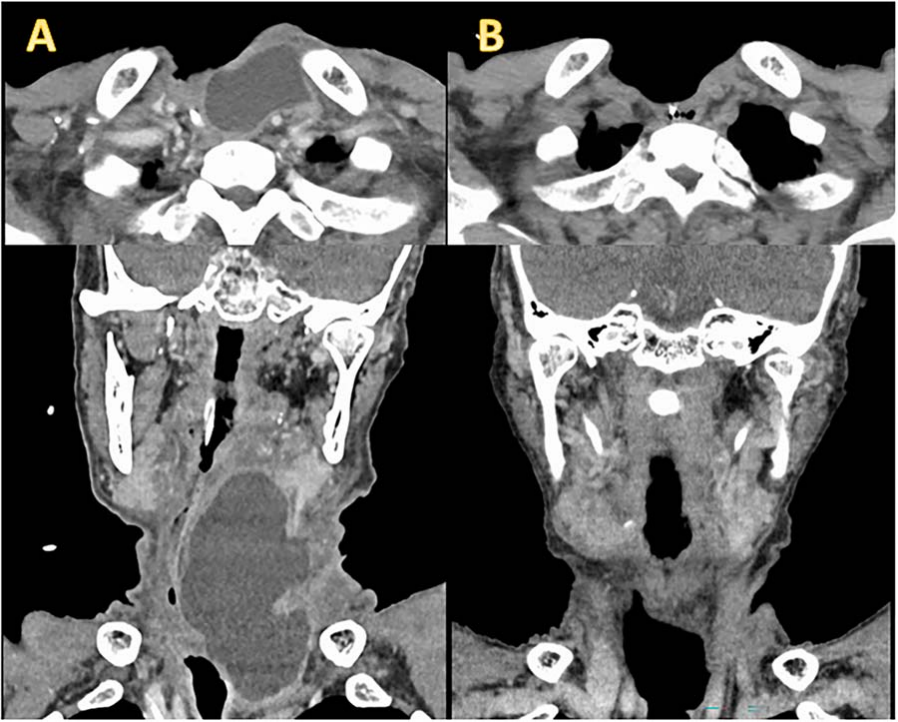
Enhanced CT before and after OK432 injection. (A) Before OK432 injection. Cervical CT shows a chylous leakage in the left supraclavicular fossa. (B) After two cycles of OK4432 injection. The chylous leakage completely disappeared after OK432 sclerotherapy.
